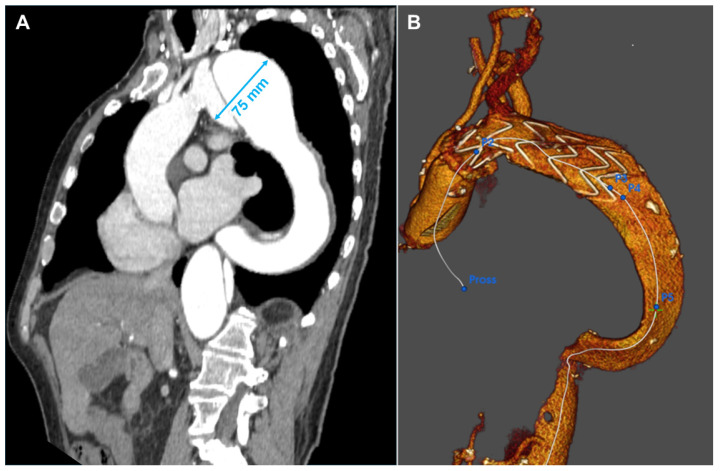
Patient 3’s Computed Tomography Angiography showing the evolution of the type B dissection with an enormous aneurysm of 75 mm in Zone 3 (A). Three-dimensional reconstruction of the result after the frozen elephant trunk operation (B). After one month, the patient was treated with another TEVAR in the descending aorta.
